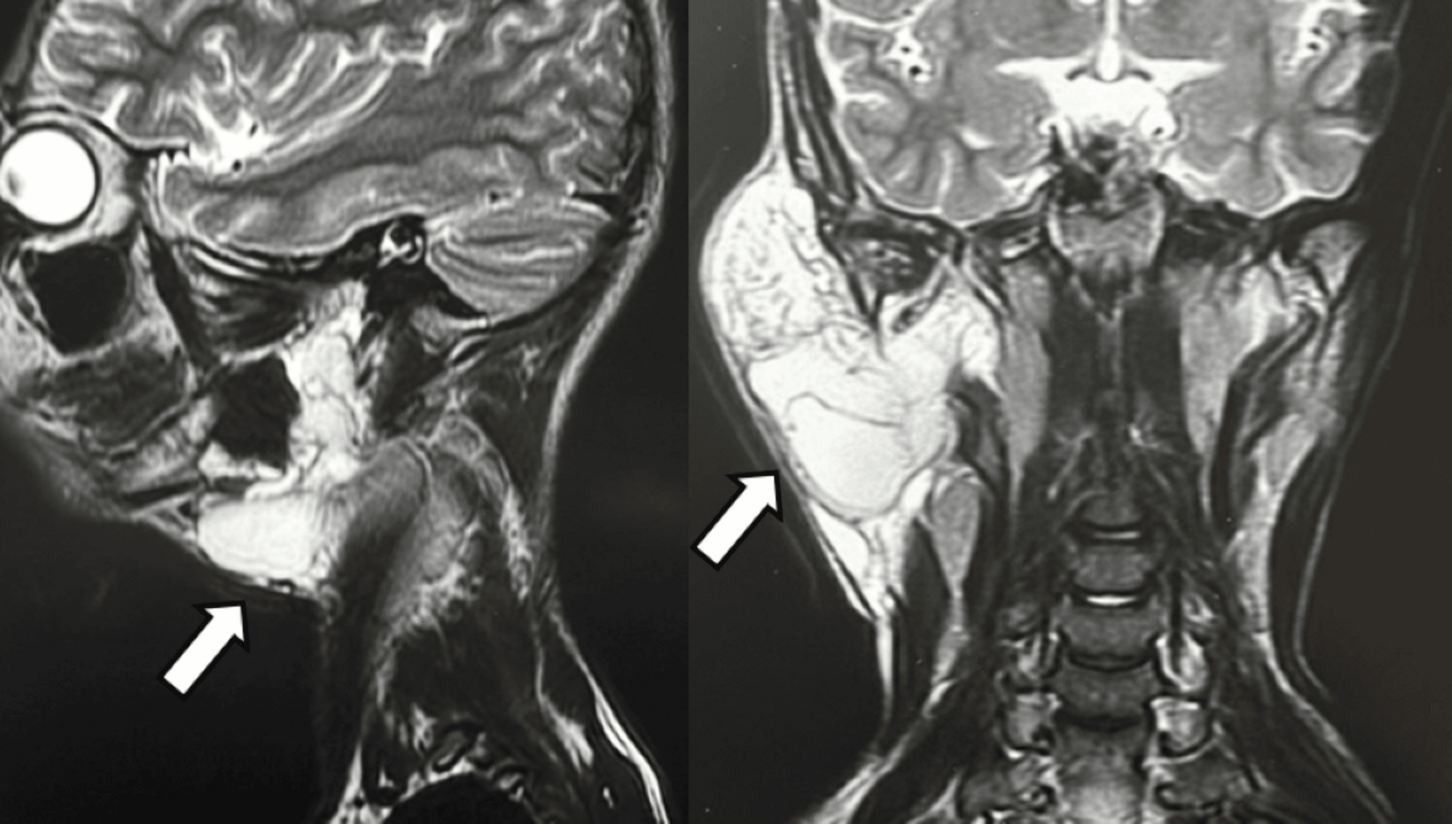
MRI showing the multiloculated, multiseptated lesion in the right infra-auricular region. MRI, magnetic resonance imaging.
